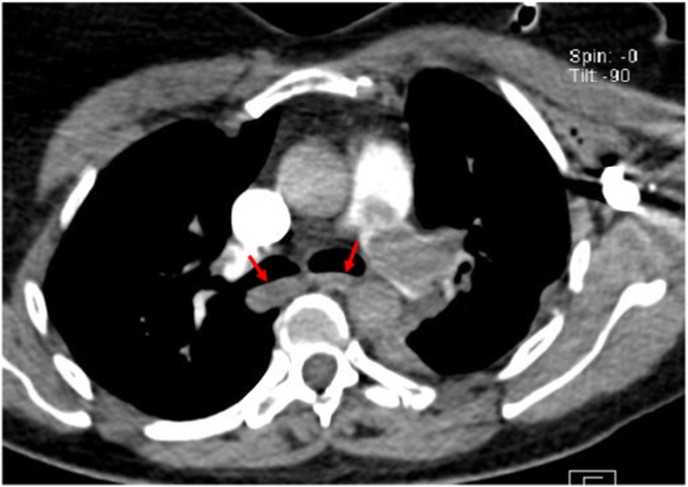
Axial view of a computed tomography pulmonary angiography showing A: pulmonary embolism in the segmental left branch of the left lobe pulmonary artery B: pulmonary embolism in the pulmonary artery trunk.
